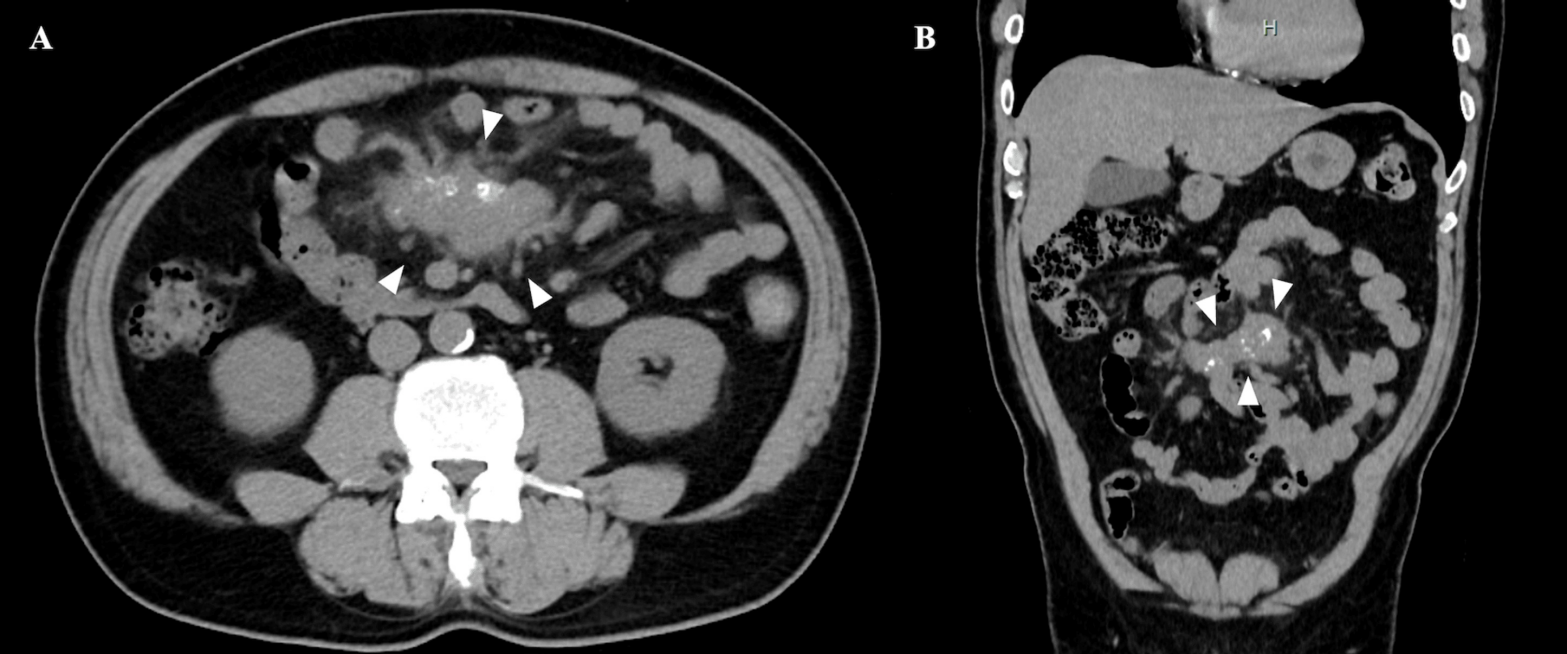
Plain abdominal computed tomography. An irregular mass, measuring 5.5 cm in length with calcification, is seen in the mesentery of the small intestine (A, horizontal section; B, coronal section). The mass is indicated by white triangles. The mesentery surrounding the lesion also shows ciliary shadows, with extensive contact with the small intestine.
